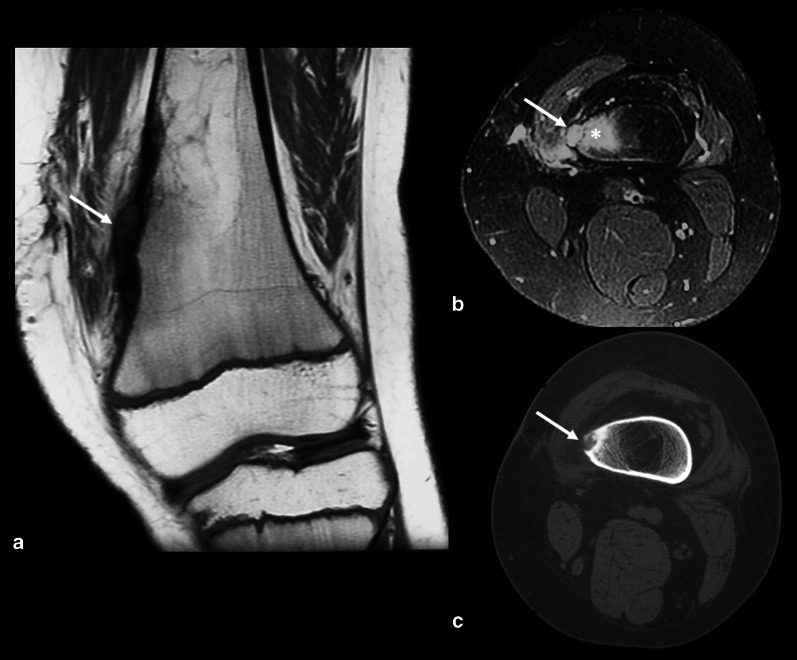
Osteoid osteoma near a growth plate. An 11–year-old male with a history of surgical removal of an OO on the distal metadiaphysis of the left femur. His symptoms persisted, and follow-up MRI (a, b) and CT (c) showed a residual nidus at the medial femoral margin represented by the intracortical nodule (arrows) and surrounding bone marrow edema (asterisk in b). In a, note that the affected side of the distal femur is longer than the lateral side, resulting in a femoral deformity and length discrepancy of the left lower limb
